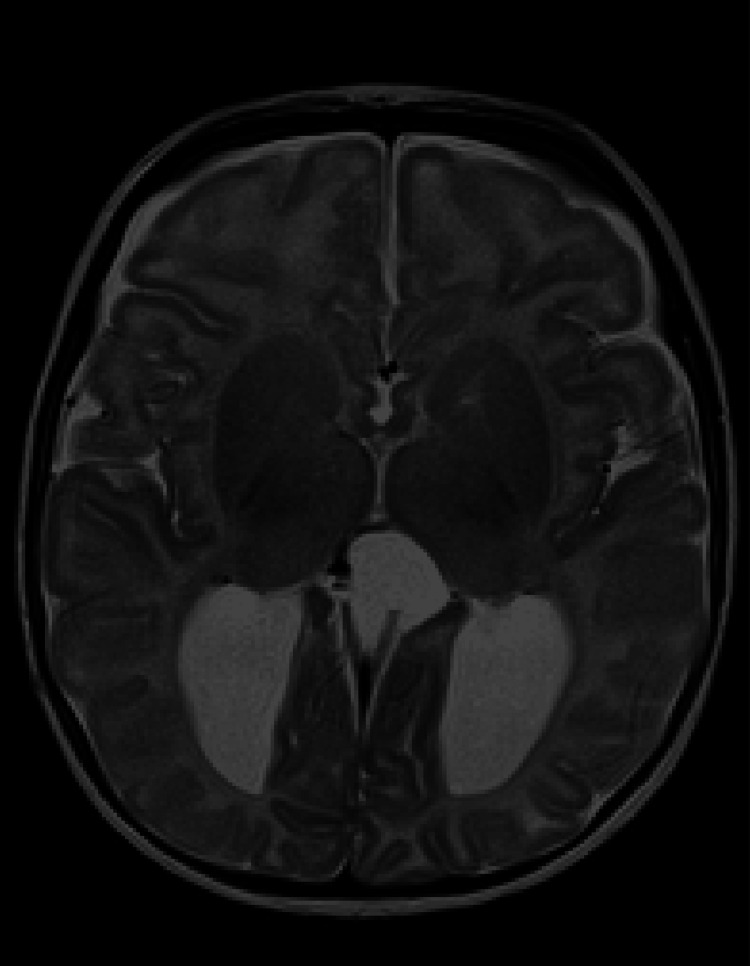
MRI of patient 2's brain (axial view). Colpocephaly with dysgenesis of the corpus callosum and third ventricle dilation and projection superiorly as a left paracentral dorsal cyst.
